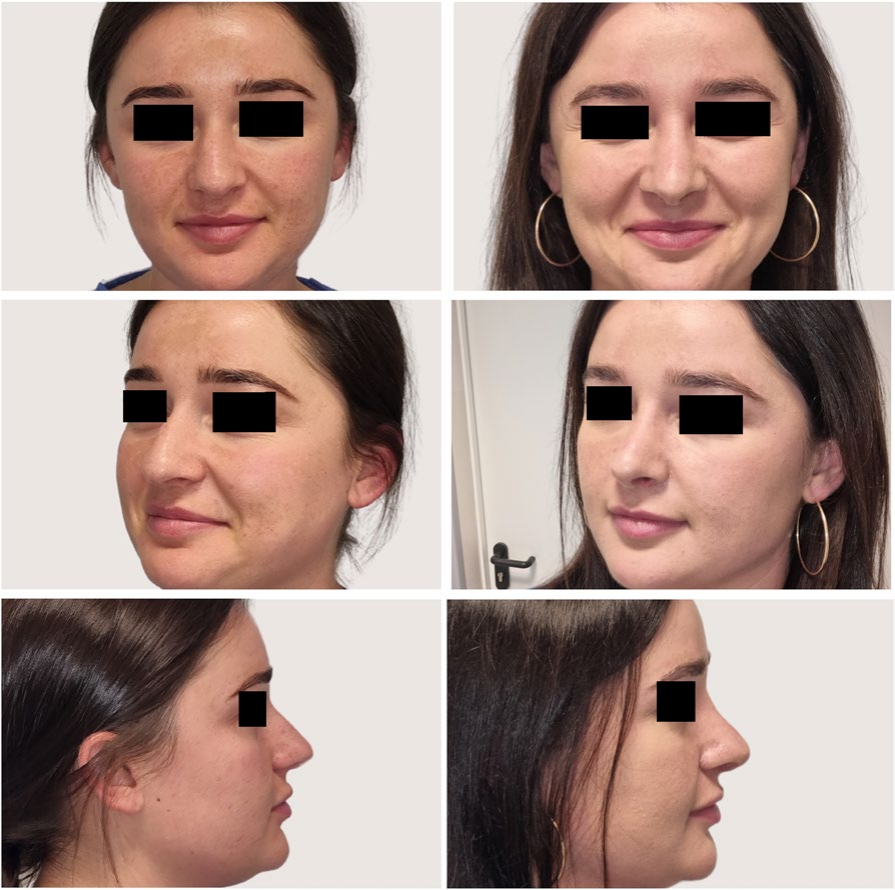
CT-assisted precision rhinoplasty in patient D desiring natural feminine harmonious aesthetic outcome in the nose with “thick” skin
